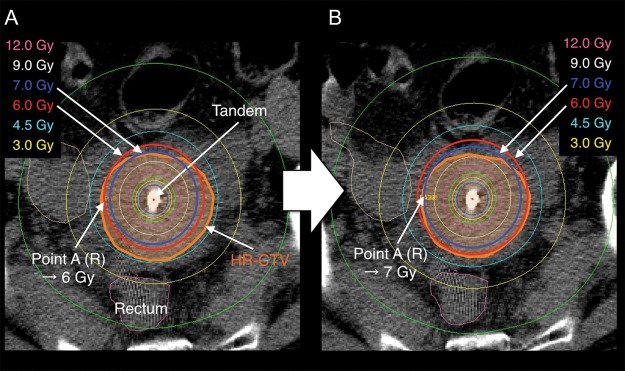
Axial computed tomography image of an example case of ICBT. (A) A dose of 6 Gy was administered to point A. The high-risk clinical target volume (HR-CTV) was not covered with the 6-Gy isodose line. (B) A dose of 7 Gy was prescribed to point A. The 6-Gy isodose line almost encompasses the HR-CTV.
